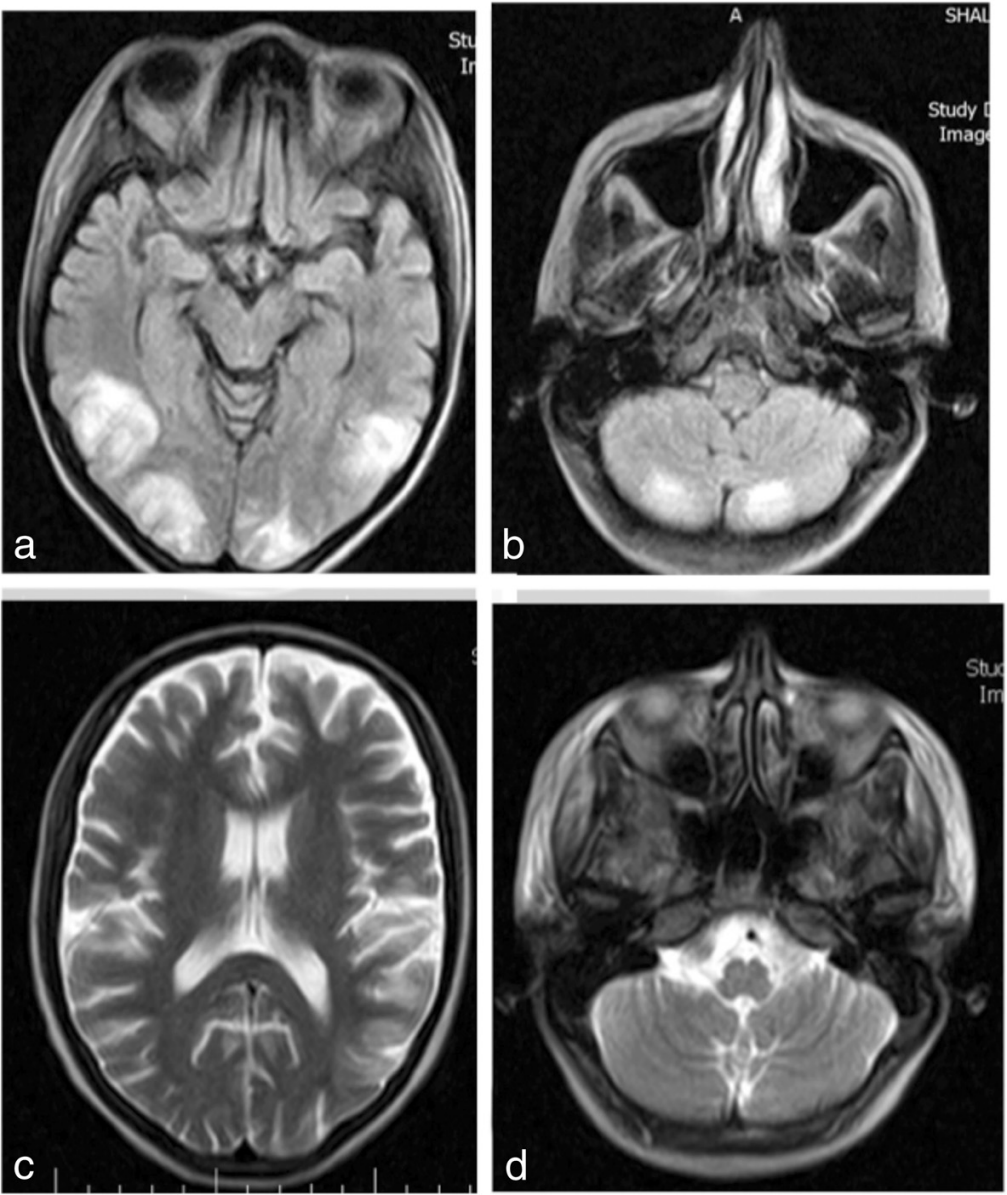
Brain magnetic resonance imaging T2-weighted/fluid-attenuated inversion recovery scan showing high signal intensities in a the subcortical white matter of occipital, posterior parietal, and posterior temporal lobes and b the cerebellum. c, d Follow-up brain magnetic resonance imaging T2-weighted/fluid-attenuated inversion recovery scan 8 days after the first imaging showed complete resolution
